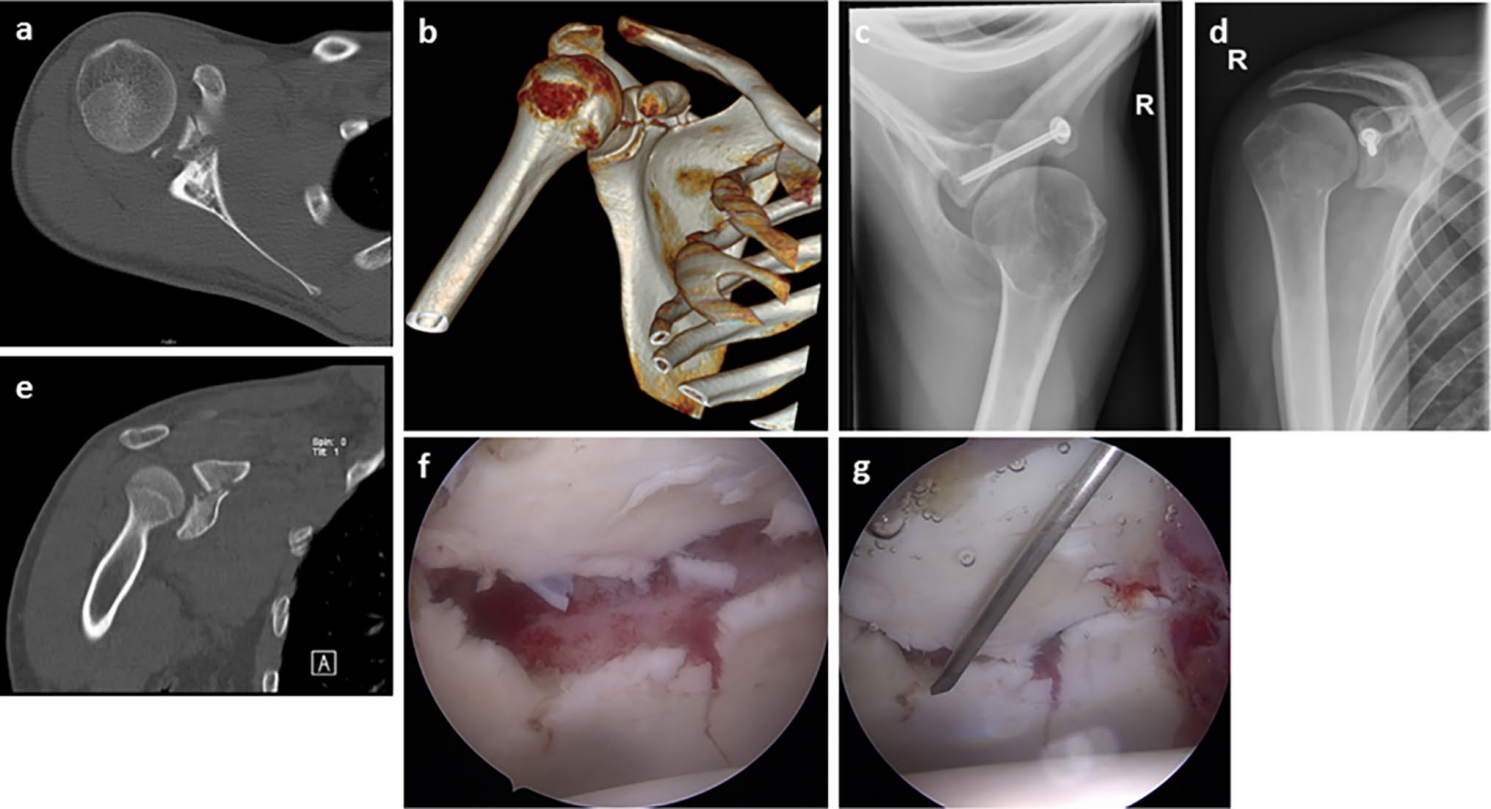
a and e CT images of a type 3b coracoid fracture; b 3D CT image of a type 3b coracoid fracture; c and d type 3b coracoid fracture with screw osteosynthesis; f and g intraoperative arthroscopic view on displaced glenoid fracture
